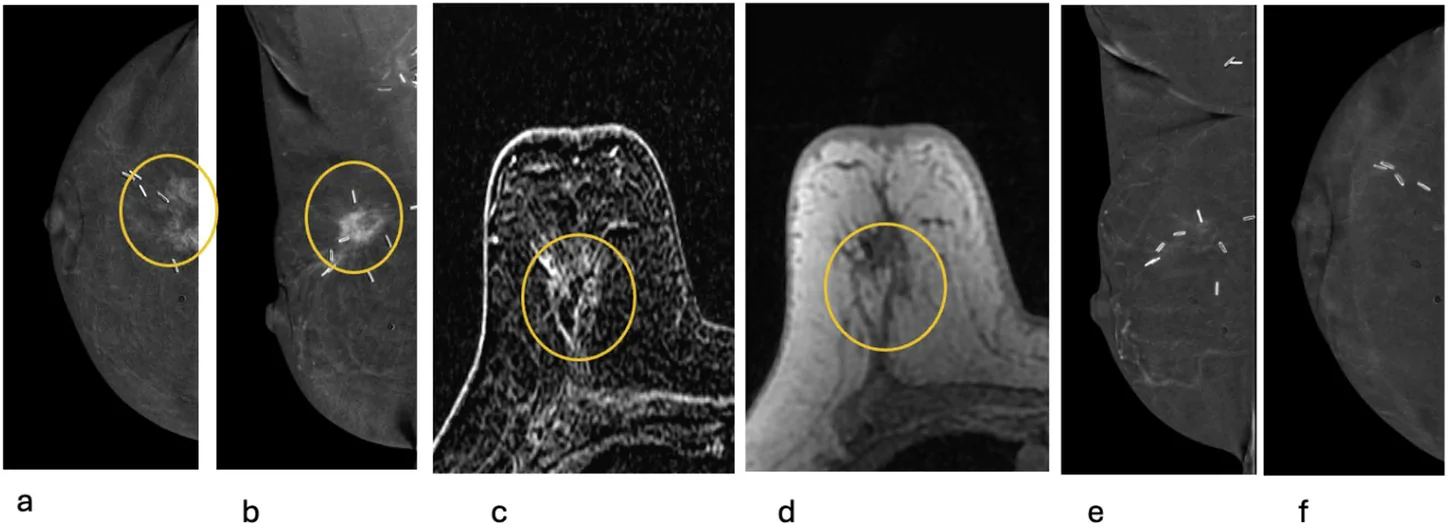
High-conspicuity fat necrosis. A 60-year-old woman with a history of right breast cancer status and lumpectomy underwent routine screening CEM. On CEM, a high-conspicuity peripherally enhancing mass is seen in the lumpectomy bed on the recombined images, on both craniocaudal (a) and mediolateral (b) projections. Subsequent contrast-enhanced MRI (c axial post-contrast subtraction sequence, and d T1-weighted non-contrast sequence) demonstrated peripheral enhancement with central fat, consistent with benign fat necrosis. Follow-up CEM at two years (e craniocaudal projection and f mediolateral oblique projection) demonstrated resolving enhancement in the lumpectomy bed, further supporting a benign etiology
